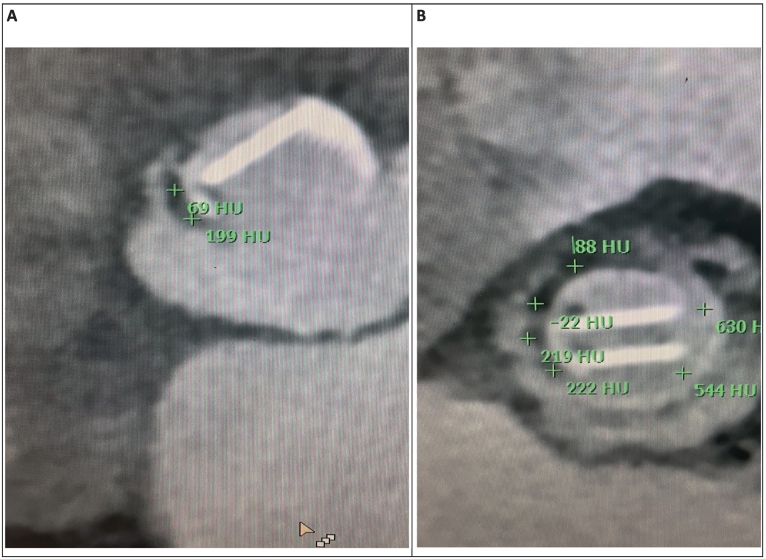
CT with contrast showed the attenuation of thrombus < 90HU surrounded by pannus formation with CT attenuation >145 HU: pre thrombolytic therapy (A) and post the single cycle of lytic therapy (B).
